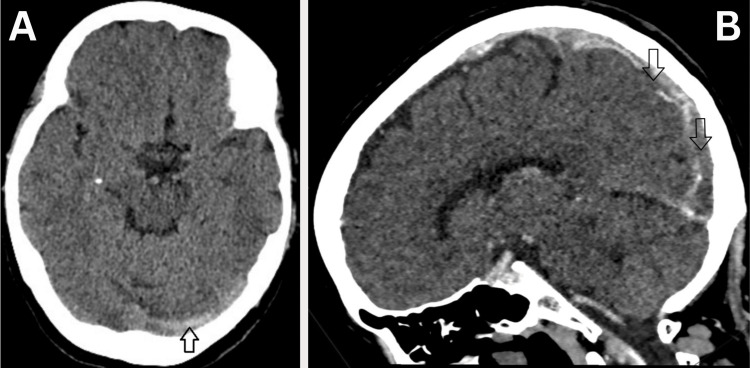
(A) Axial non-contrast CT head demonstrating hyperdensity within the superior sagittal sinus and cortical veins (arrow), consistent with acute cerebral venous sinus thrombosis (CVST). (B) Sagittal CT venogram showing filling defects in the superior sagittal sinus (arrows), consistent with CVST.
